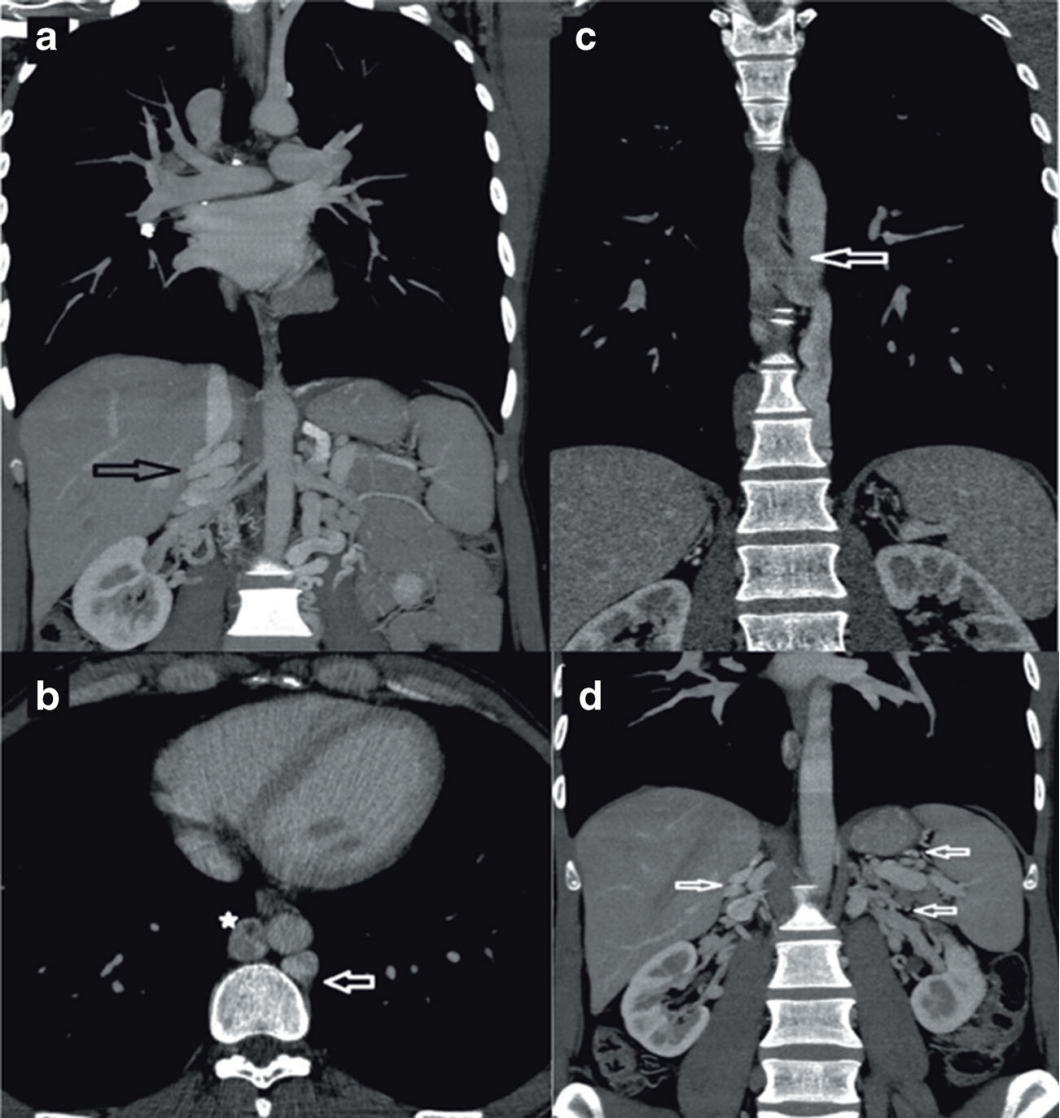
a Coronal MIP MDCT image showing an appearance of complete thrombosis of the infrarenal portion of IVC (on the right (*arrow*) and tortuous appearance of gonadal vein (*median pathway*) (on the *left*) (*asterisk*) b Coronal MIP MDCT image showing enlarged inferior hemorrhoidal vein (*portal pathway*) (*arrow*) c Sagittal MIP MDCT image showing ascending lumbar veins with an appearance of pseudomasses at the level of lumbar vertebra (*deep pathway*) (*arrow*) d Coronal MPR MRI image showing infrarenal IVC agenesis (arrow) (e) Coronal MPR MRI image showing enlarged epigastric vein (*superficial pathway*) (*arrow*)
